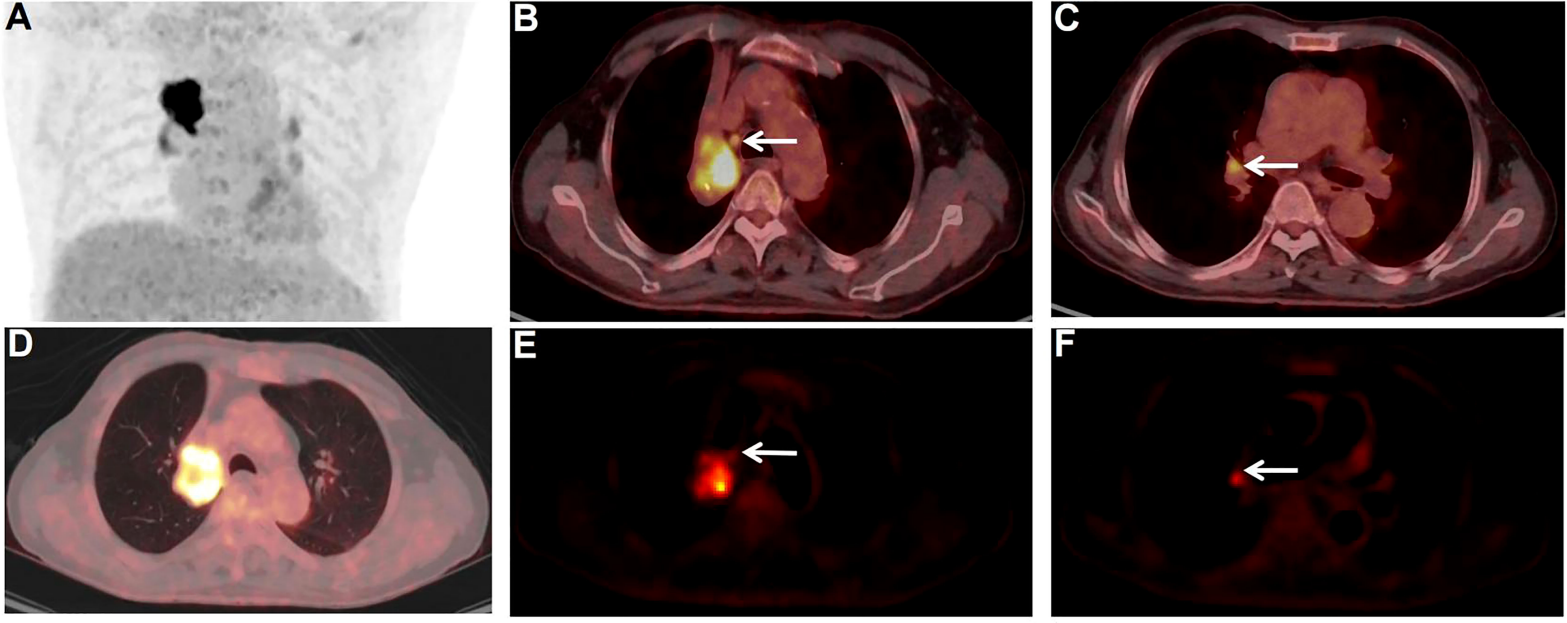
PET/CT images of non-metastatic and metastatic FDG-avid LNs. A 77-year-old male patient. Surgical pathology confirmed a squamous cell carcinoma in the upper lobe of the right lung. PET/CT scan showed multiple FDG-avid LNs in the mediastinal region and pulmonary hilar region. The FDG-avid LN (E, white arrow) in zone 4R (B, white arrow) of the mediastinal region was pathologically confifirmed to be cancer-free, with a size of 0.7×0.6 cm, SUVmax of 4.0, and Ki of 0.0084 ml/g/min. The other FDG-avid LN (F, white arrow) in zone 11R (C, white arrow) of the pulmonary hilar region was pathologically proven to be metastases, with a size of 0.7×0.7 cm, SUVmax of 4.9, and Ki of 0.0202 ml/g/min.
